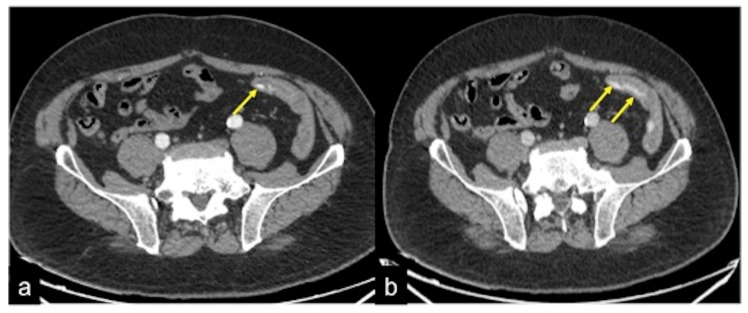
CT multiphasic study of bleeding at the proximal tract of sigma. In the arterial axial image after contrast media administration (a) it is possible to detect a blush of active bleeding (arrow). In the venous phase (b) a dimensional increase of the hyperdense blush is present (arrows) as a sign of active incremental bleeding in the venous phase.
